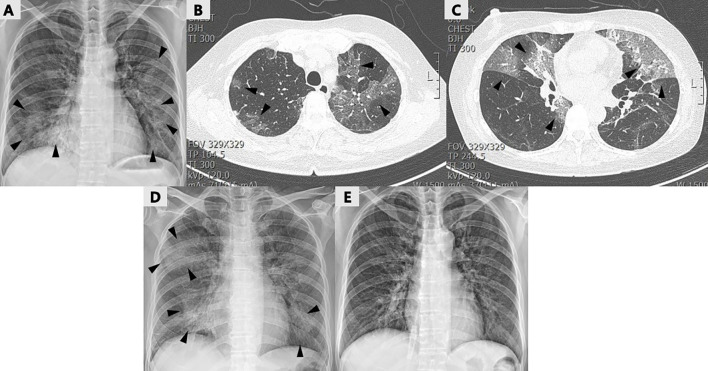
Radiologic progression and clinical response to intravenous immunoglobulin (IVIG). (A) Chest radiograph showing multifocal consolidations in both lungs. (B, C) Axial chest CT images showing bilateral patchy consolidations and GGOs with interstitial thickening. (D) Follow-up chest radiograph showing worsening multifocal consolidations and GGOs, accompanied by fever during hospitalization, prompting initiation of IVIG. (E) Chest radiograph at discharge showing significant resolution of lung lesions after IVIG treatment.
